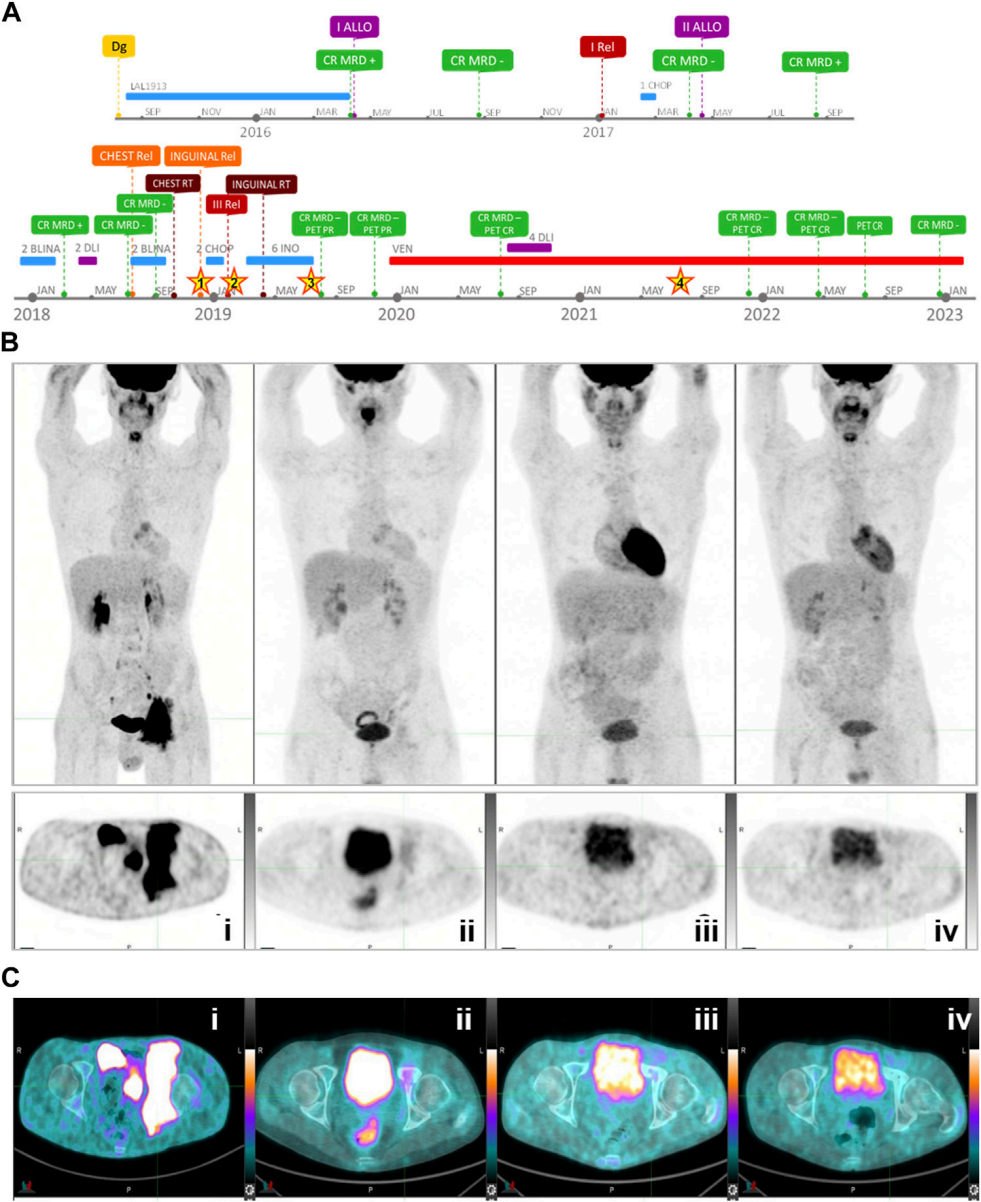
(A) Timeline of patient’s leukemia medical history (Dg, diagnosis; ALLO, allogeneic transplantation; MRD, minimal residual disease; CR, complete response; Rel, relapse; DLI, donor lymphocyte infusion; RT, radiotherapy; BLINA, blinatunomab; INO, inotuzomab ozogamicin; PET PR, partial response; VEN, venetoclax; “Stars” indicate the four analyzed time points (1: extramedullary relapse #1—Dec/2018; 2: third relapse #2—Jan/2019; 3: post-inotuzomab ozogamicin hematological remission #4—Jun/2019; 4: post-venetoclax remission—Jul/2021). (B) Whole-body (upper panel) and axial scan (lower panel) 18F-FDG PET at four time points: at relapse, in December 2018 (i); after six cycles of inotuzumab ozogamicin followed by radiotherapy, in November 2019 (ii); after 6 months of treatment with venetoclax, in July 2020 (iii), and after 27 months of treatment with venetoclax and four DLIs, in April 2022 (iv). (C) 18F-FDG PET images (axial scan) at four time points showing i) inguinal pelvic mass at relapse - SUV 9.5 (December 2018); ii) partial resolution—SUV 2.9/Deuville 3 (November 2019) reached after treatment with inotuzumab ozogamicin and radiotherapy; iii) complete resolution—SUV 1.8 (July 2020—Deuville 1) after 6 months of venetoclax, and iv) after 27 months of continuative treatment with venetoclax and four DLIs—SUV 1.4 (April 2022—Deuville 1).
